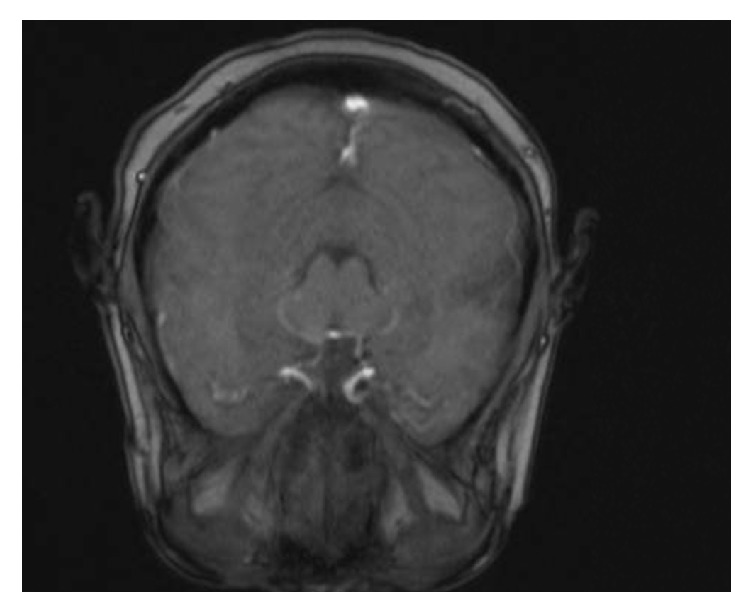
Magnetic resonance imaging of the brain mass. The image shows a small enhancing parasagittal mass.
